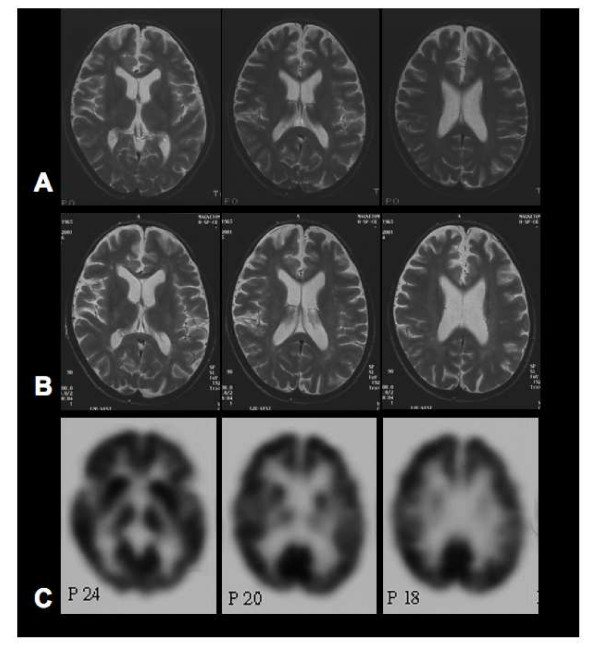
Imaging studies. (A) Initial magnetic resonance imaging revealed signs of cerebral atrophy with a prominent inter-hemispheral fissure at the age of 23 years. (B) The second magnetic resonance imaging 11 years later did not reveal any progression of cerebral atrophy nor any other signs of cystinosis-associated encephalopathy. (C) Positron emission tomography at the age of 34 years demonstrated normal cortical glucose utilisation without signs of encephalopathy.
